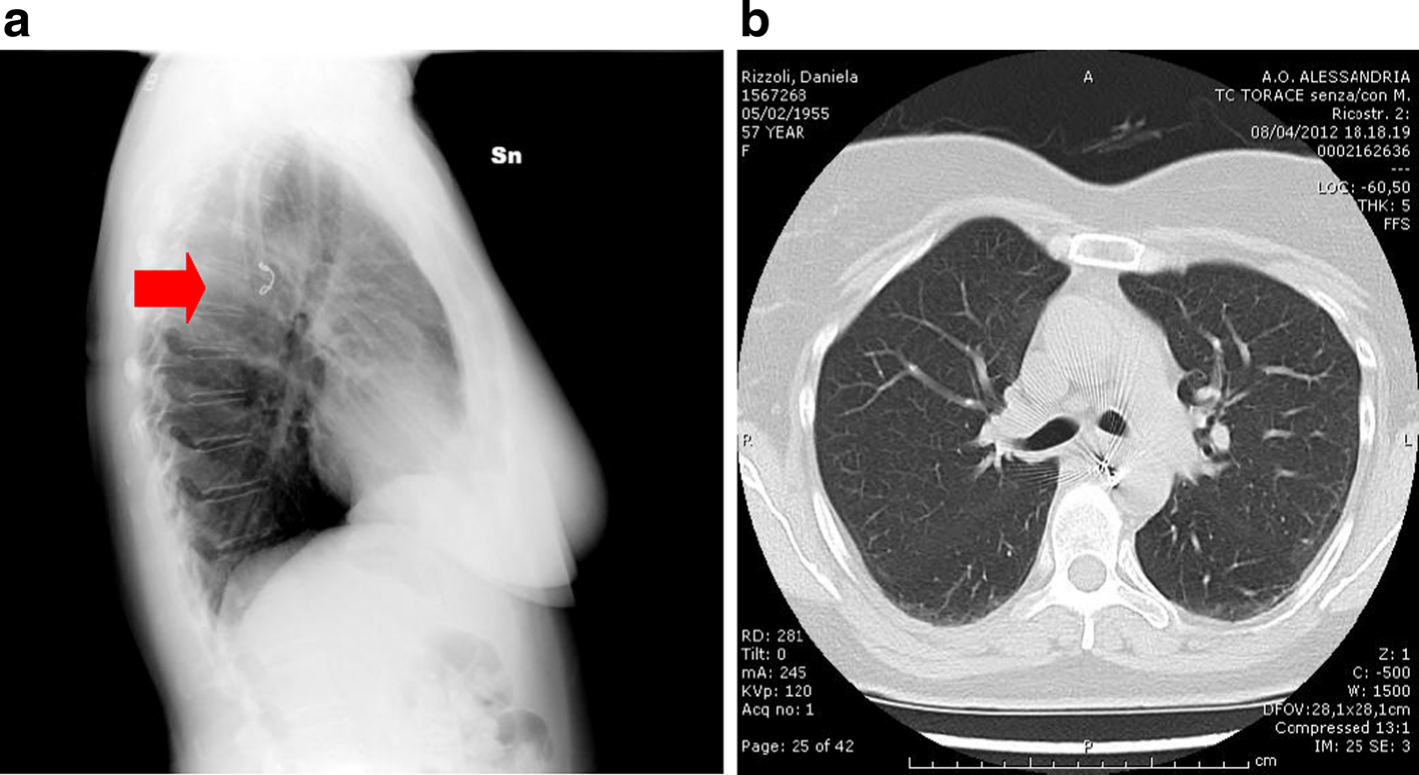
(Black arrow): Spiral in the right bronchial artery, [a: X-ray b: CT].
